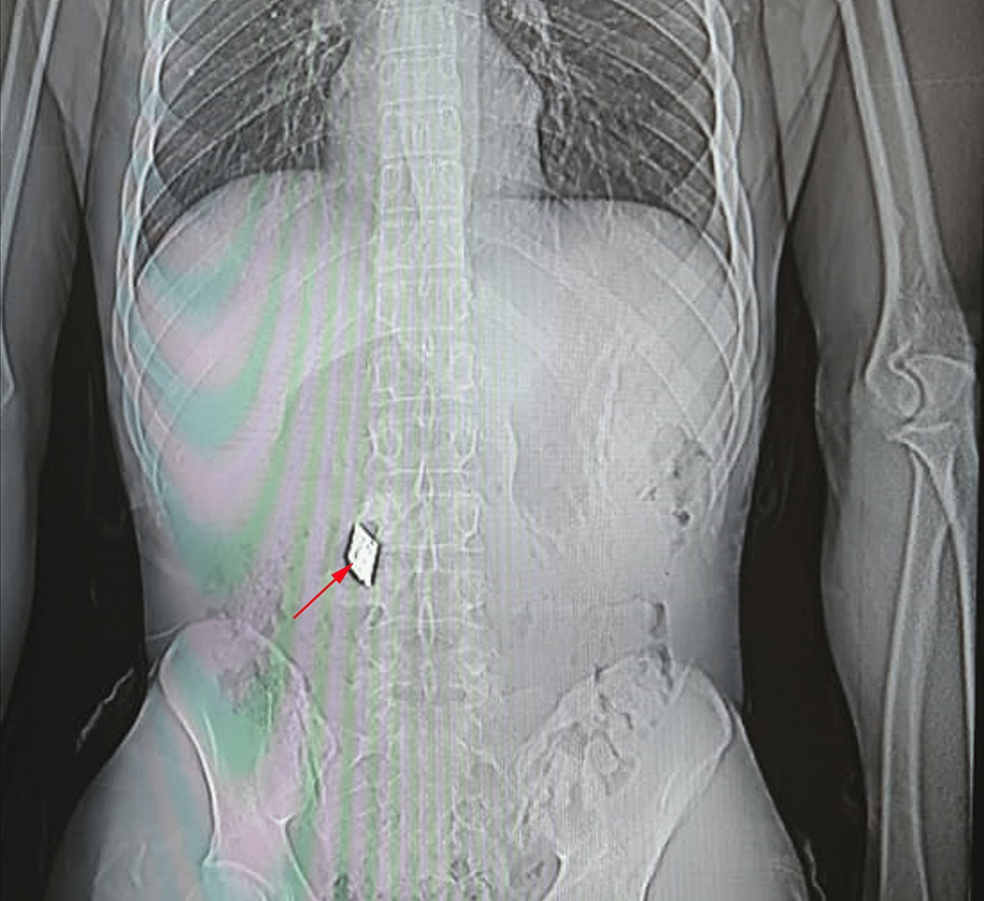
Abdominal radiograph The image reveals the presence of a 50 x 20 mm metallic foreign body (red arrow) at the right lumbar area at the level of L3 and L4 vertebral bodies.
